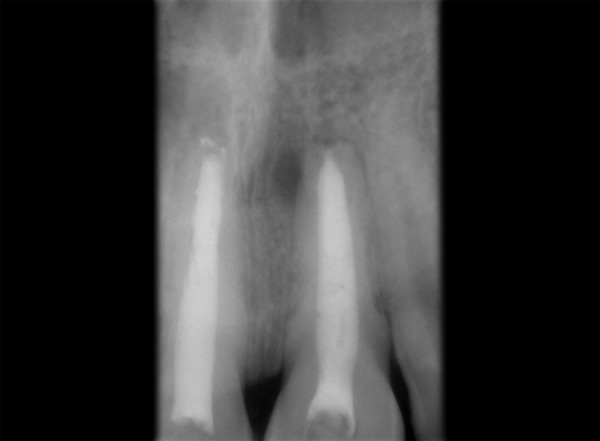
Radiographic appearance at 1 year 6 months
